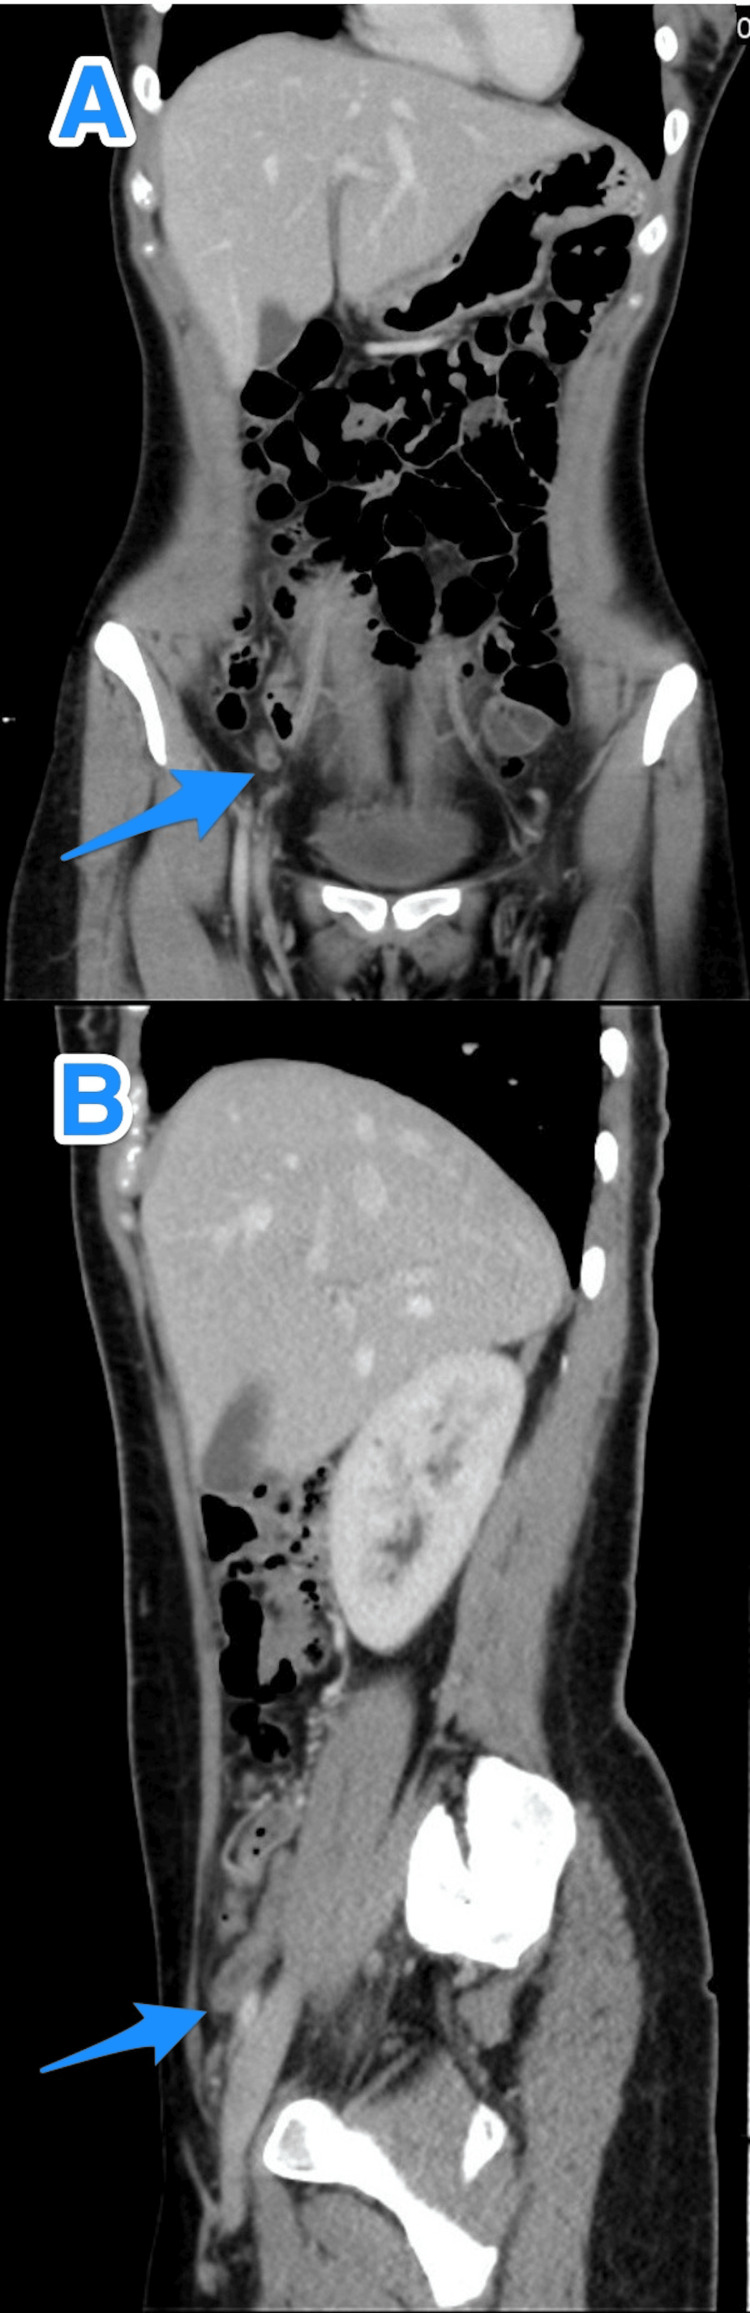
A multiview computed tomography abdomen scan (A and B) shows a small diverticulum close to the tip of an inflamed appendix (arrows).
